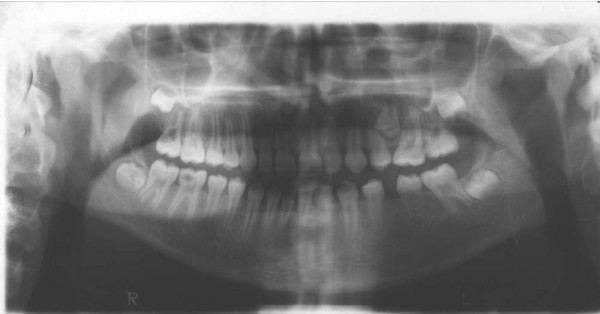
Pretreatment panoramic radiograph of the patient.
